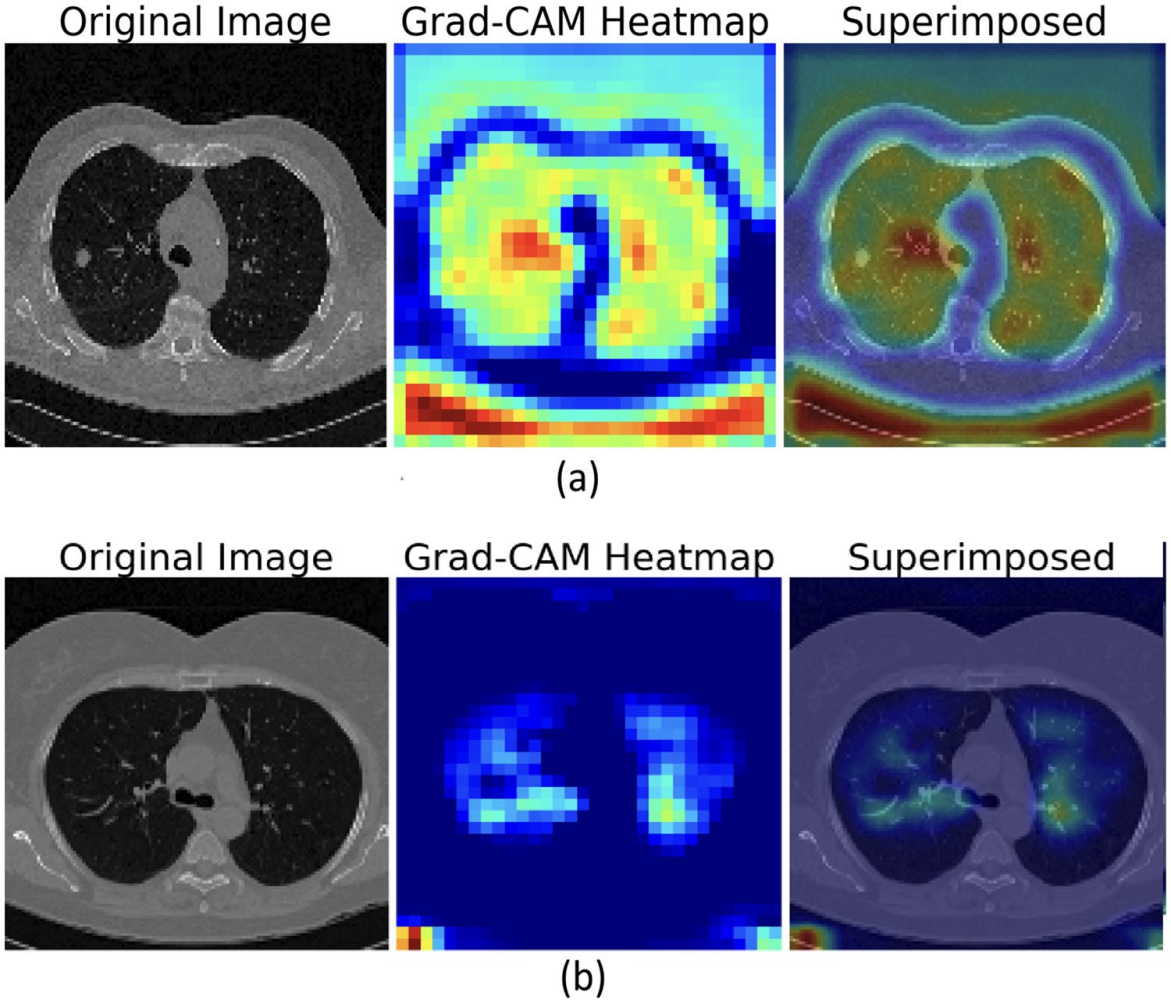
Sample of CT lung Image With XAI (Grad-CAM), (a) CT lung image Abnormal, (b) CT lung image Normal.
